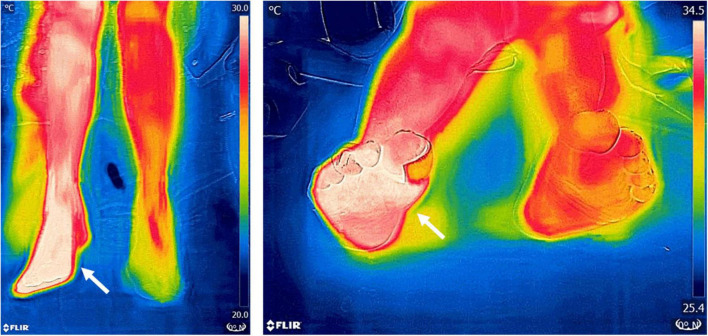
Thermographic imaging of a patient after endovascular revascularization. Thermograms 24 h post-surgery of a patient with severe peripheral artery disease (PAD) who underwent endovascular revascularization show a positive gradient of temperature of 4.2°C compared to the non-revascularized limb. The hotspots (arrows) are highly predictive of a successful treatment. In this patient, reperfusion was confirmed angiographically during surgery and by Doppler ultrasound at follow-up. Images, courtesy of JA-C, were acquired using a FLIR One Pro mobile camera.
